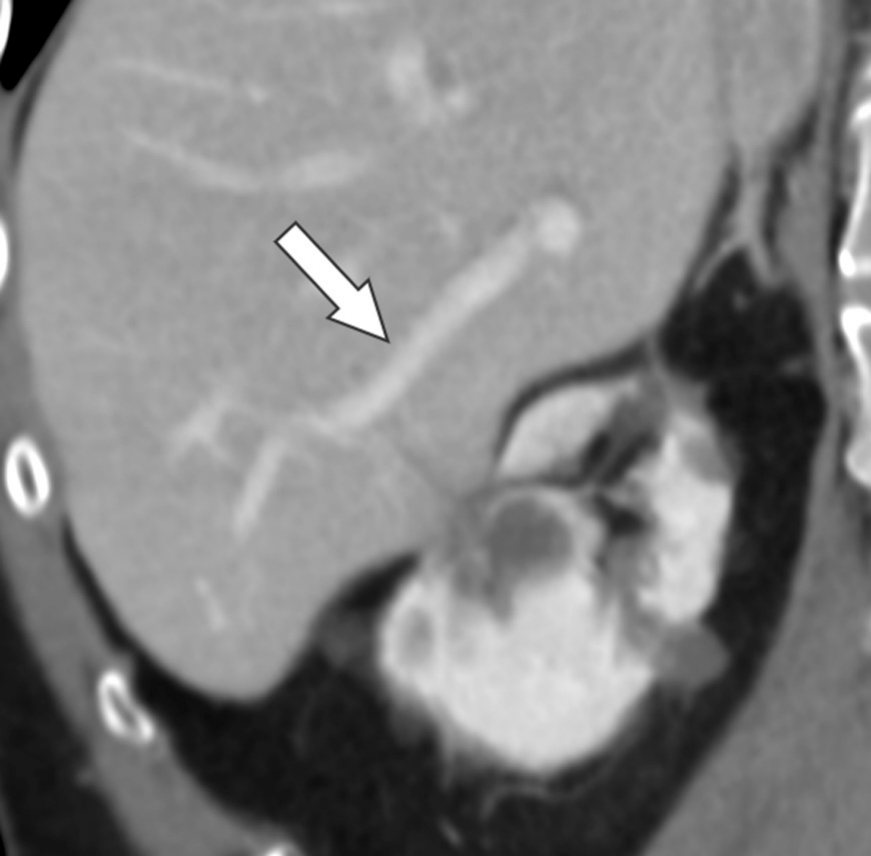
Oblique coronal contrast-enhanced CT before cryoablation reveals the normal liver parenchyma and patent portal vein branch (arrow).
